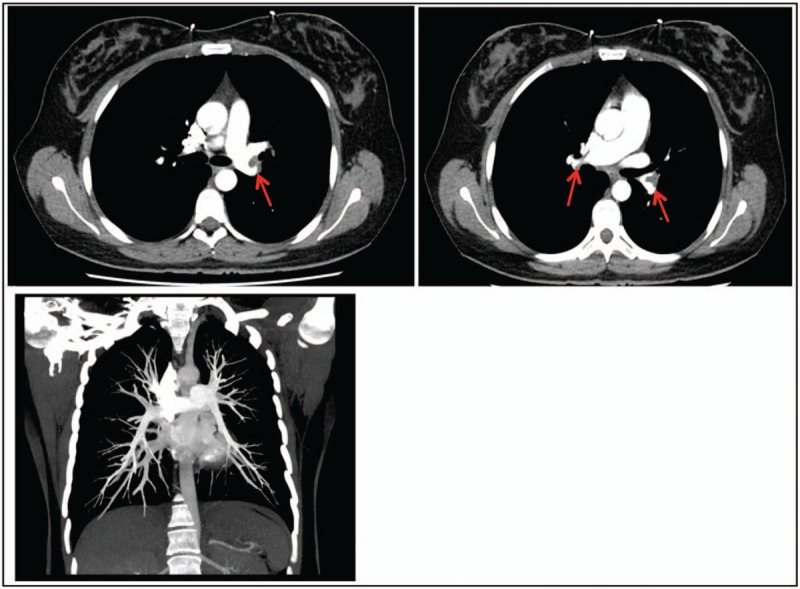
Contrast-enhanced computed tomography of the chest after thrombolysis, *red arrows* in the first column showed thrombi occupying the pulmonary arteries bilaterally before discharge, and the recanalization of the lung perfusion in the second column was observed after 3 months follow-up.
